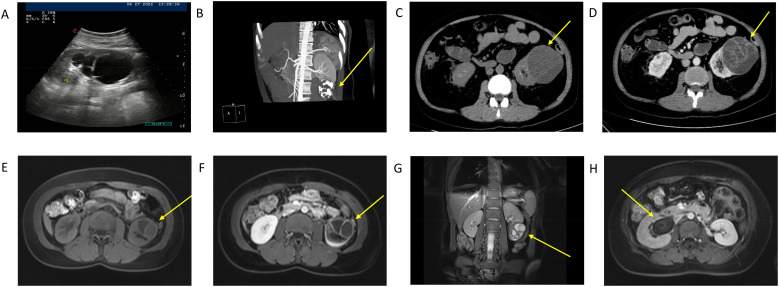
Radiological images: (A) (case 1): color doppler ultrasonography of the kidney. The ultrasound reveals liquid anechoic structures within the renal parenchyma, with slightly thick cyst walls and irregular grid-like septations observed inside the cysts; (B) (case 5): Renal artery computed tomography angiography (CTA) showing the mass at the lower pole of the kidney. Enhanced scanning of the renal artery reveals punctate enhancement within the mass; (C, D) (case 4): Computed tomography plain scan and contrast-enhanced imaging of cystic nephroma. Plain CT scans indicate that single oval-shaped low-density lesions are present within the kidneys. (E–G) (case 5): T1-weighted magnetic resonance imaging (MRI), T1-weighted contrast-enhanced MRI, and T2-weighted MRI of cystic nephroma. MRI examination shows that the cystic lesions exhibit low signal intensity on T1-weighted images and high signal intensity on T2-weighted images. Some patients present linear septations with intermediate to low signal intensity on T1-weighted images. Upon contrast enhancement, linear septations or capsular enhancements are visible; (H) (case 6): T1-weighted contrast-enhanced MRI demonstrating tumor-induced compression of the renal pelvis and calyces.
